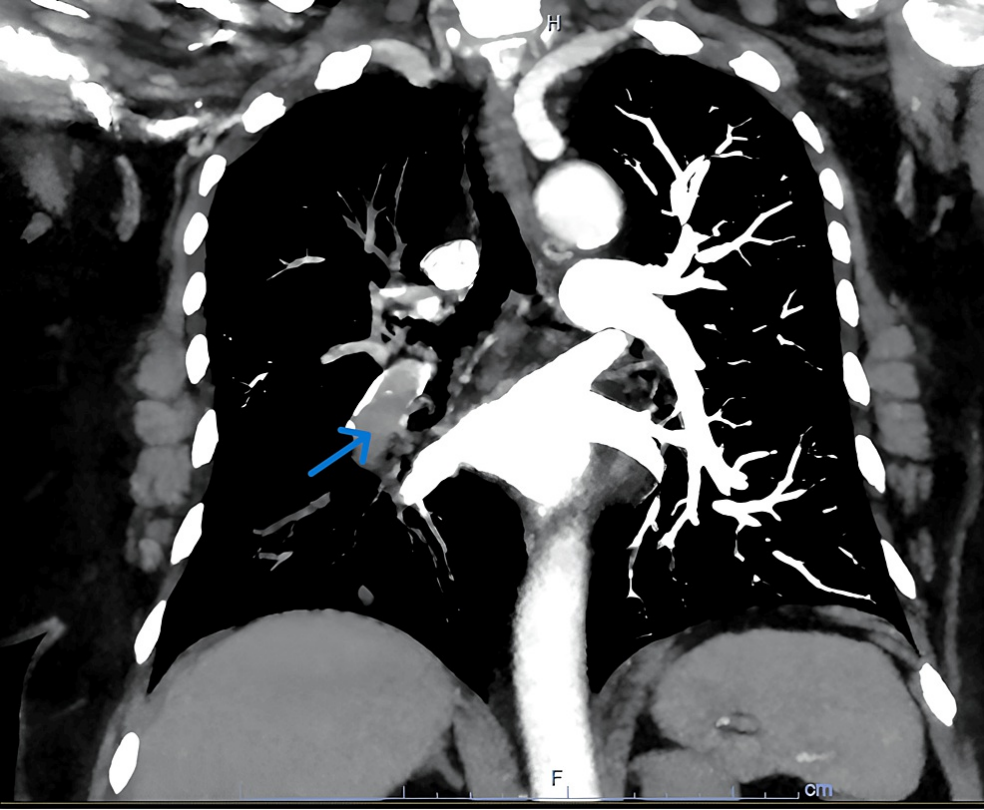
CT pulmonary embolism protocol Arrow showing a filling defect in the right main/interlobar pulmonary arteries representing a pulmonary embolism
